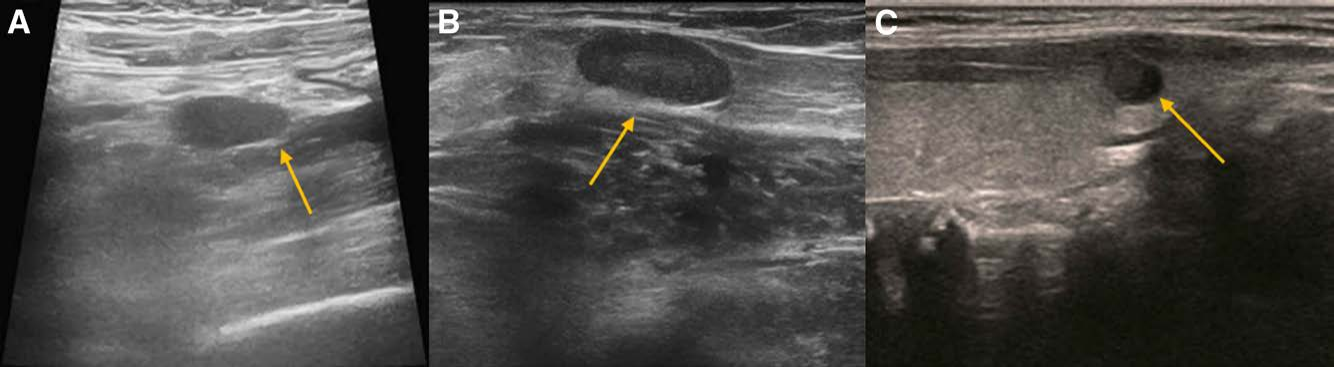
(A) Ultrasound of the retroperitoneal lymph nodes shows enlargement of the left iliac vessels-adjacent lymph node, approximately 2.0 × 0.9 cm (yellow arrow). (B) Ultrasound of the inguinal lymph nodes shows enlargement of multiple lymph nodes, with the largest on the right, approximately 1.2 × 0.6 cm (yellow arrow). (C) Ultrasound of the cervical and axillary lymph nodes shows enlargement of multiple lymph nodes, with the largest on the right, approximately 2.3 × 0.9 cm (yellow arrow).
